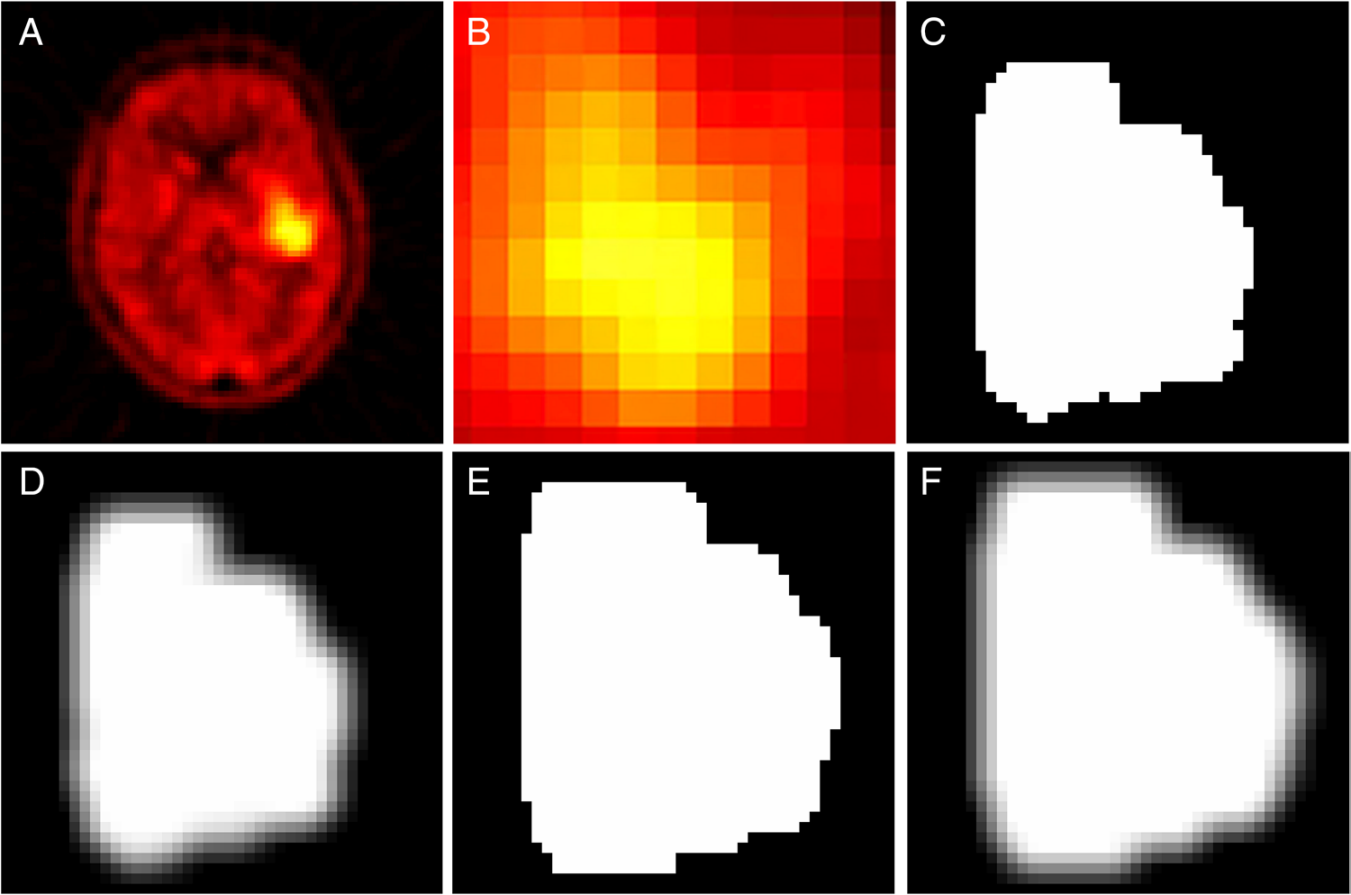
A Axial view of an example lesion in the Glioma cohort (MET-PET, Hot iron palette with palette ranges 0–6 SUV). B Magnified axial view of the tumor C Reference binary delineation (Ref-B). D Reference fuzzy delineation (Ref-F). E Extended binary delineation (Ext-B). F Extended Fuzzy delineation (Ext-F). MET—methionine; PET—positron emission tomography; SUV—standard uptake value. Note that images C-F are delineated masks with 2 × 2 × 2 mm resampling for the radiomic analysis
